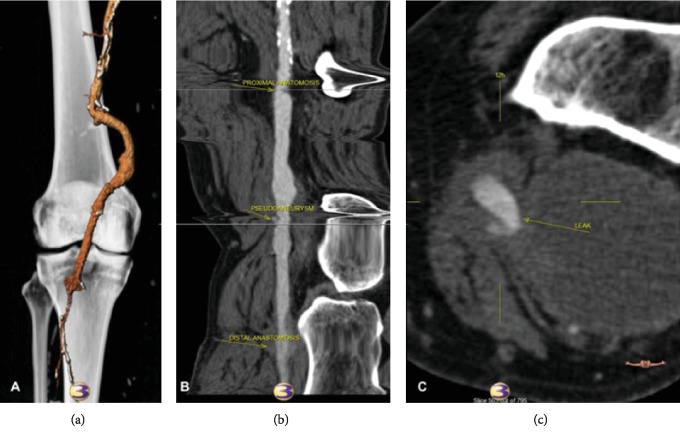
(a) 3D CT scan reconstruction showed a pseudoaneurysm of the popliteal-popliteal artery vein bypass graft. (b) Centre lumen line reconstruction for venous bypass graft pseudoaneurysm evaluation. (c) Pseudoaneurysm of the venous bypass graft with a 7.2 × 5 cm of maximum diameter.
